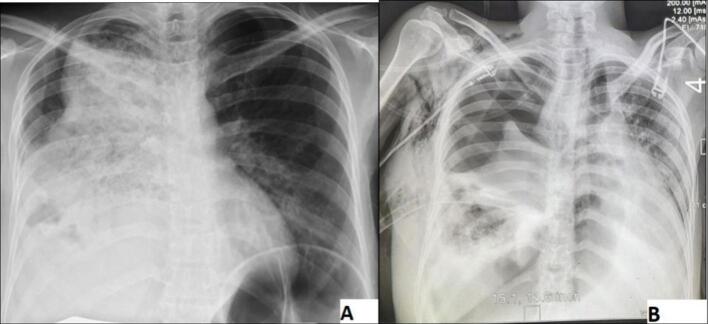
(A) An anteroposterior chest X-ray revealed right middle and lower zone opacification, obscured right heart border, and blunted cost phrenic angle and (B) a control chest x ray showing Chest tube insitu, partial lung re-expansion, and reduced pleural opacity.
